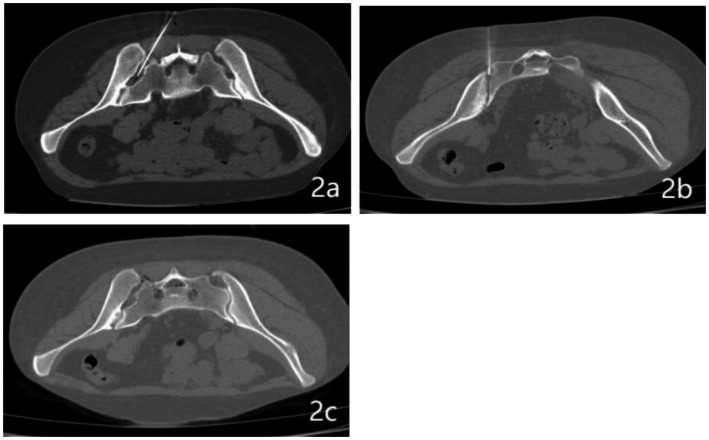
CT-guided joint cavity release surgery. (A) Puncture of the left sacroiliac joint cavity utilizing a specialized puncture needle. (B) Engagement of the posterior ramus of the sacroiliac joint via puncture needle insertion. (C) Detailed visualization of gas distribution both within the sacroiliac joint cavity and its adjacent regions.
